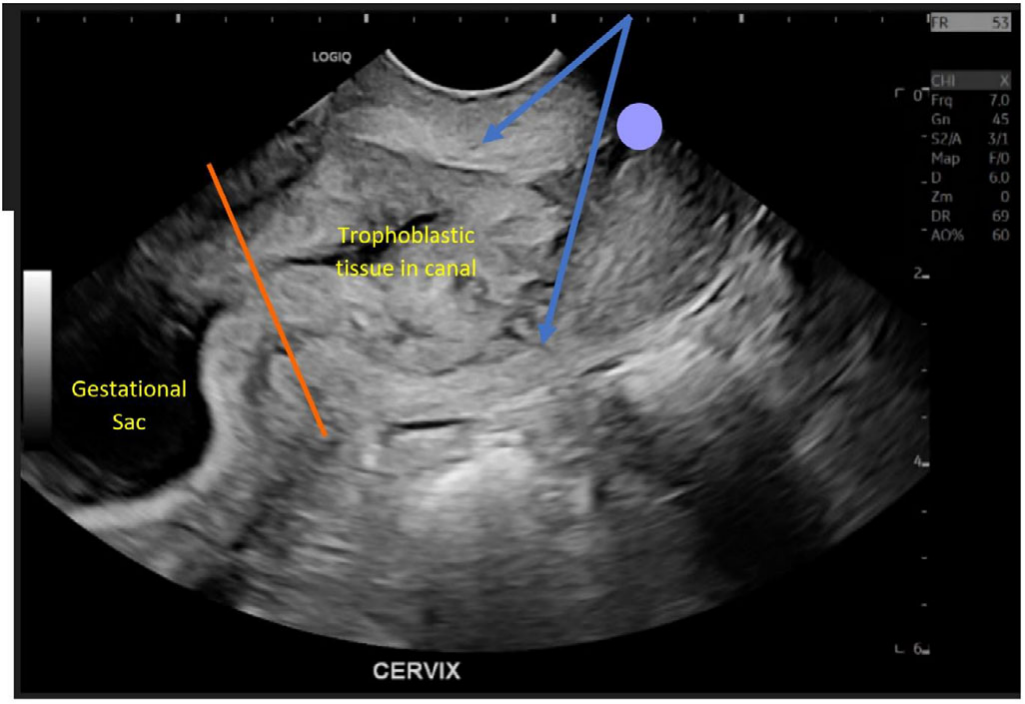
Outpatient ultrasound at 6 weeks 6 days of gestation demonstrates sagittal view of the lower uterine segment and the upper cervical canal (orange line marks the internal cervical os). The cervical canal contains echogenic tissue continuous with the gestational sac. Cervical stroma can be seen around the echogenic tissue (blue arrows), and the external cervical os appears closed (purple dot). Extension of vascular echogenic tissue into the cervical canal that is continuous with the gestational sac should be considered a red flag feature to prompt further investigation to exclude differentials such as cervical ectopic pregnancy. This finding was not commented upon in the report, nor were any colour images of the endocervical canal contents supplied.
